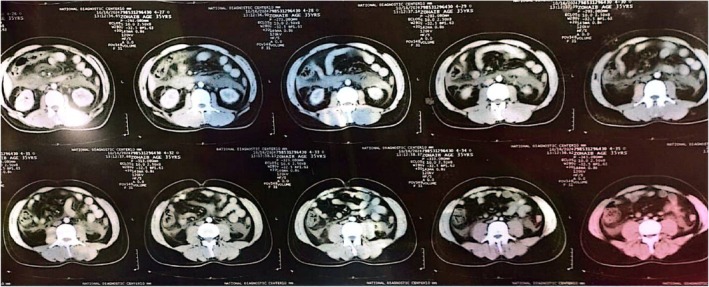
Axial Abdominal CT Scan (Upper Section) Showing an edematous pancreas with significant peripancreatic fluid collections and fat stranding.
